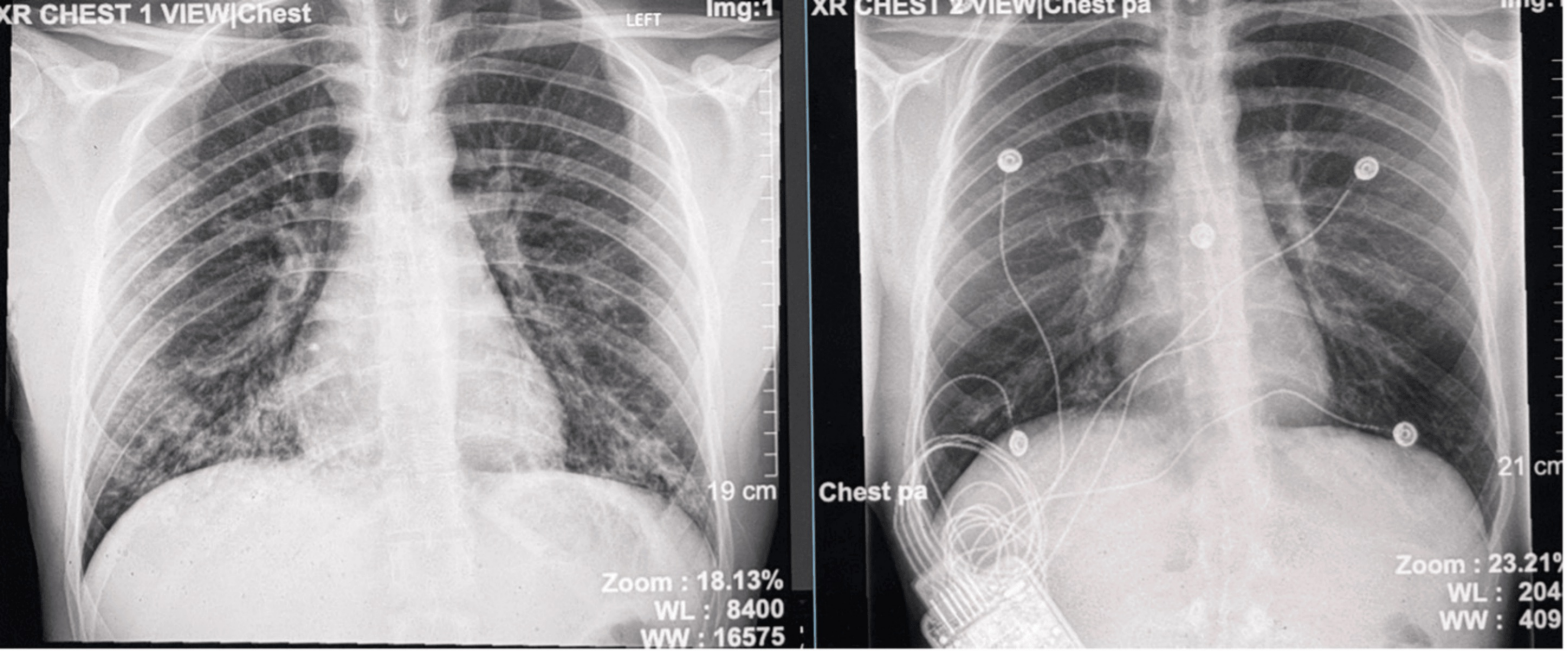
Chest X-ray images. A. Chest X-ray on admission. B. Chest X-ray prior to discharge.
